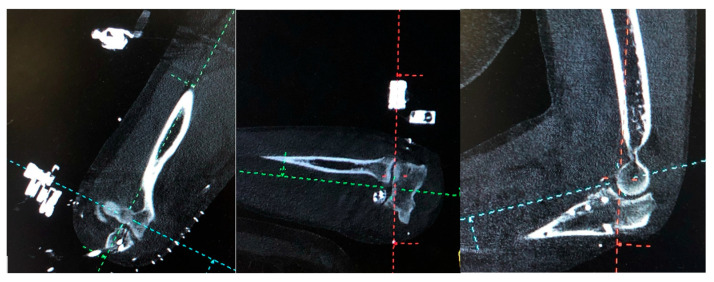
CT-3D in three projections with respect to the center of rotation of the elbow. Red line: external fixator center of rotation. Green line: elbow center of rotation.
